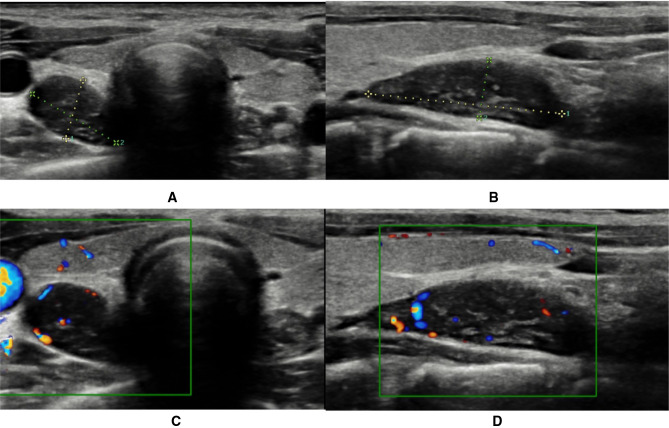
Ultrasound (A–B) and color Doppler ultrasound (C–D) images of parathyroid lesion.
